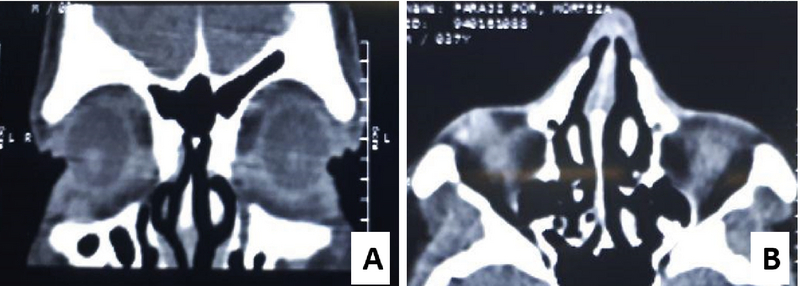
Orbital CT scan shows a well-defined mass in the inferotemporal extraconal space of the right orbit which has a single lobe posteriorly and changes to bilobed anteriorly. (A) Coronal view of the anterior orbital space. (B) Axial view of the inferior orbital space.
